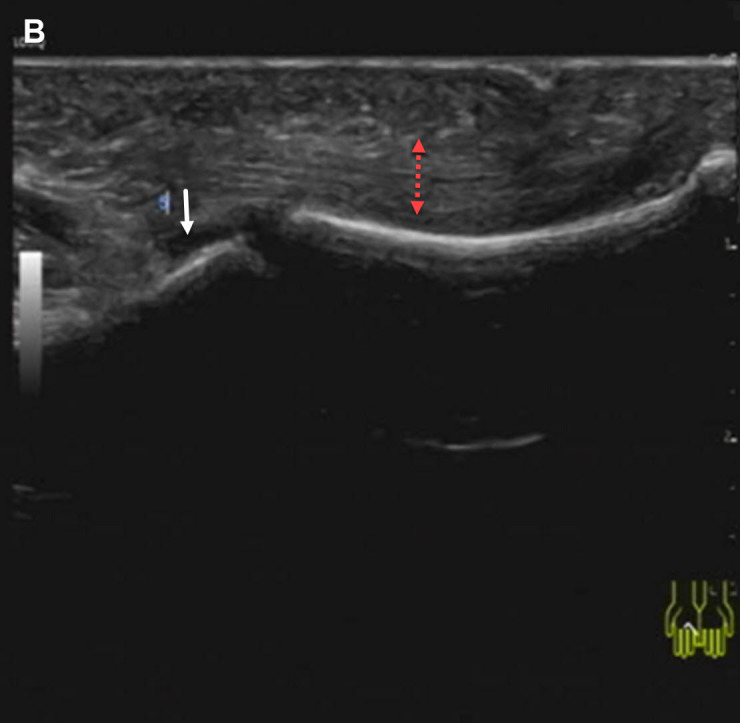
Ultrasound findings: Marked fascial thickening (5.2 mm) and mild joint effusion (arrows)
